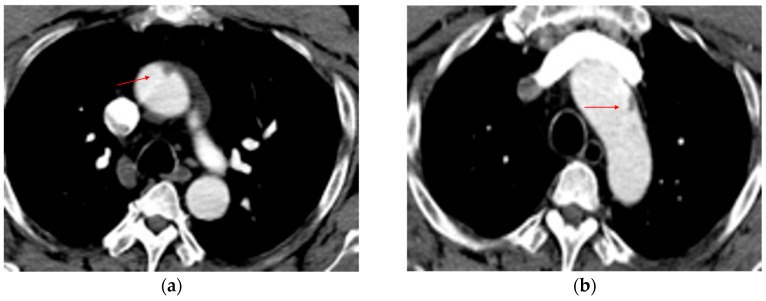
Intimal flap. (a,b) Axial CTA images show a small filling defect adherent to the aortic wall (red arrows).
